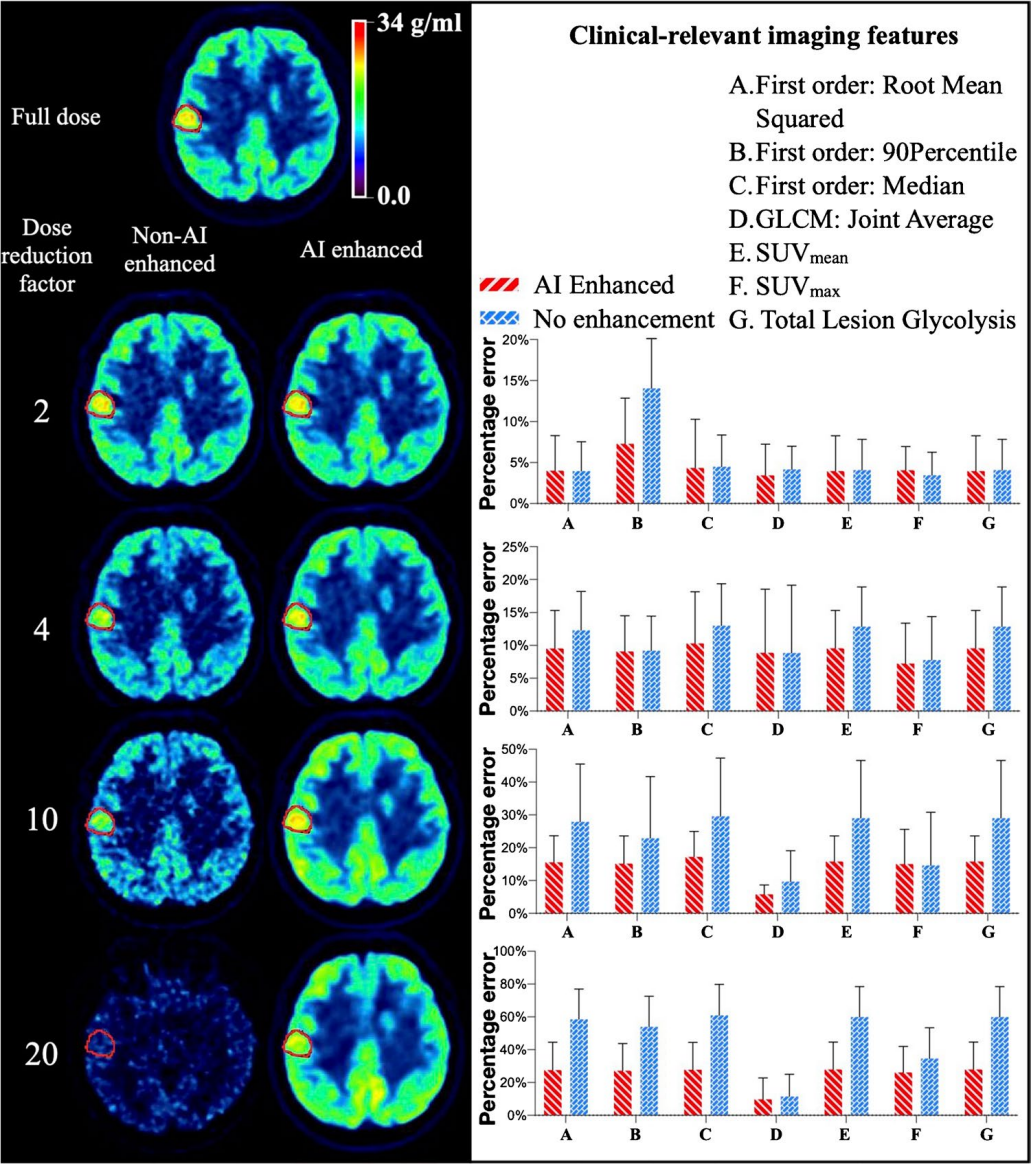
Clinical features analysis on DMI (GE, Discovery MI) with [^18^F]FDG. Results show that the enhanced images preserve an overall better quality in terms of those features and that the improvement compared to non-enhanced data tends to increase with higher DRF
